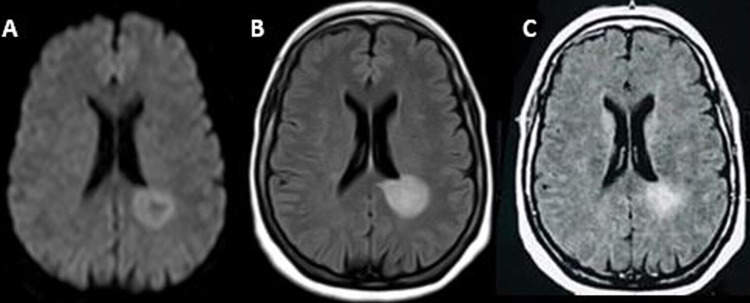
Brain magnetic resonance imaging (1.5 Tesla) showed two pseudo-nodular lesions of the peri-ventricular white matter, hyperintense in T2 and T2 flair, isointense in T1, with diffusion restriction, associated with other small lesions of the white matter on the left frontal level, right insular and bilateral occipital, enhanced after injection of gadolinium (A) Diffusion axial view; (B) T2 fluid-attenuated inversion recovery (FLAIR) axial view; (C) T1 axial view with gadolinium.
